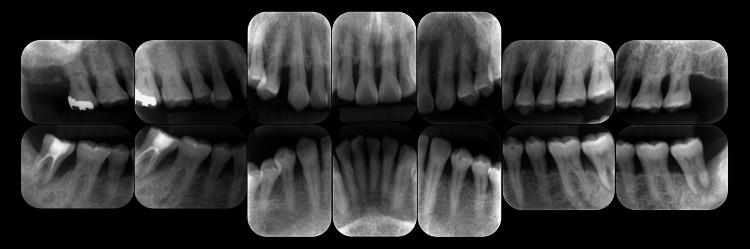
Radiographic view at the first visit
